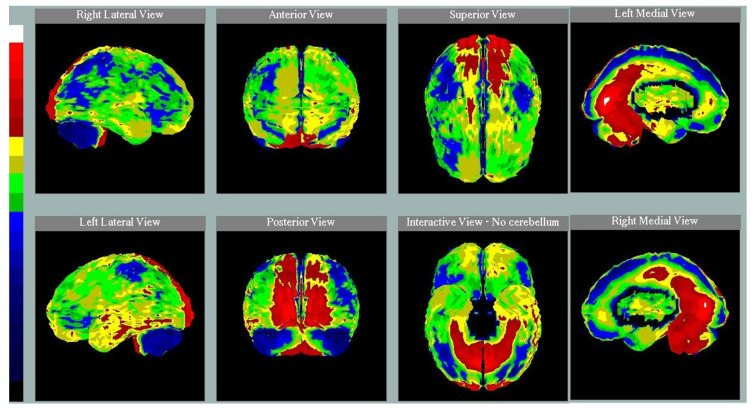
Brain perfusion SPECT with 99mTc-HMPAO in a 55-year-old woman with behavioral variant of frontotemporal dementia, Addenbrook’s Cognitive Examination—Revised score 67, 6 years of education, and total anosognosia score 29, showing hypoperfusion in inferior temporal cortex, anterior cingulate cortex, and inferior frontal gyrus, mainly of the right hemisphere.
